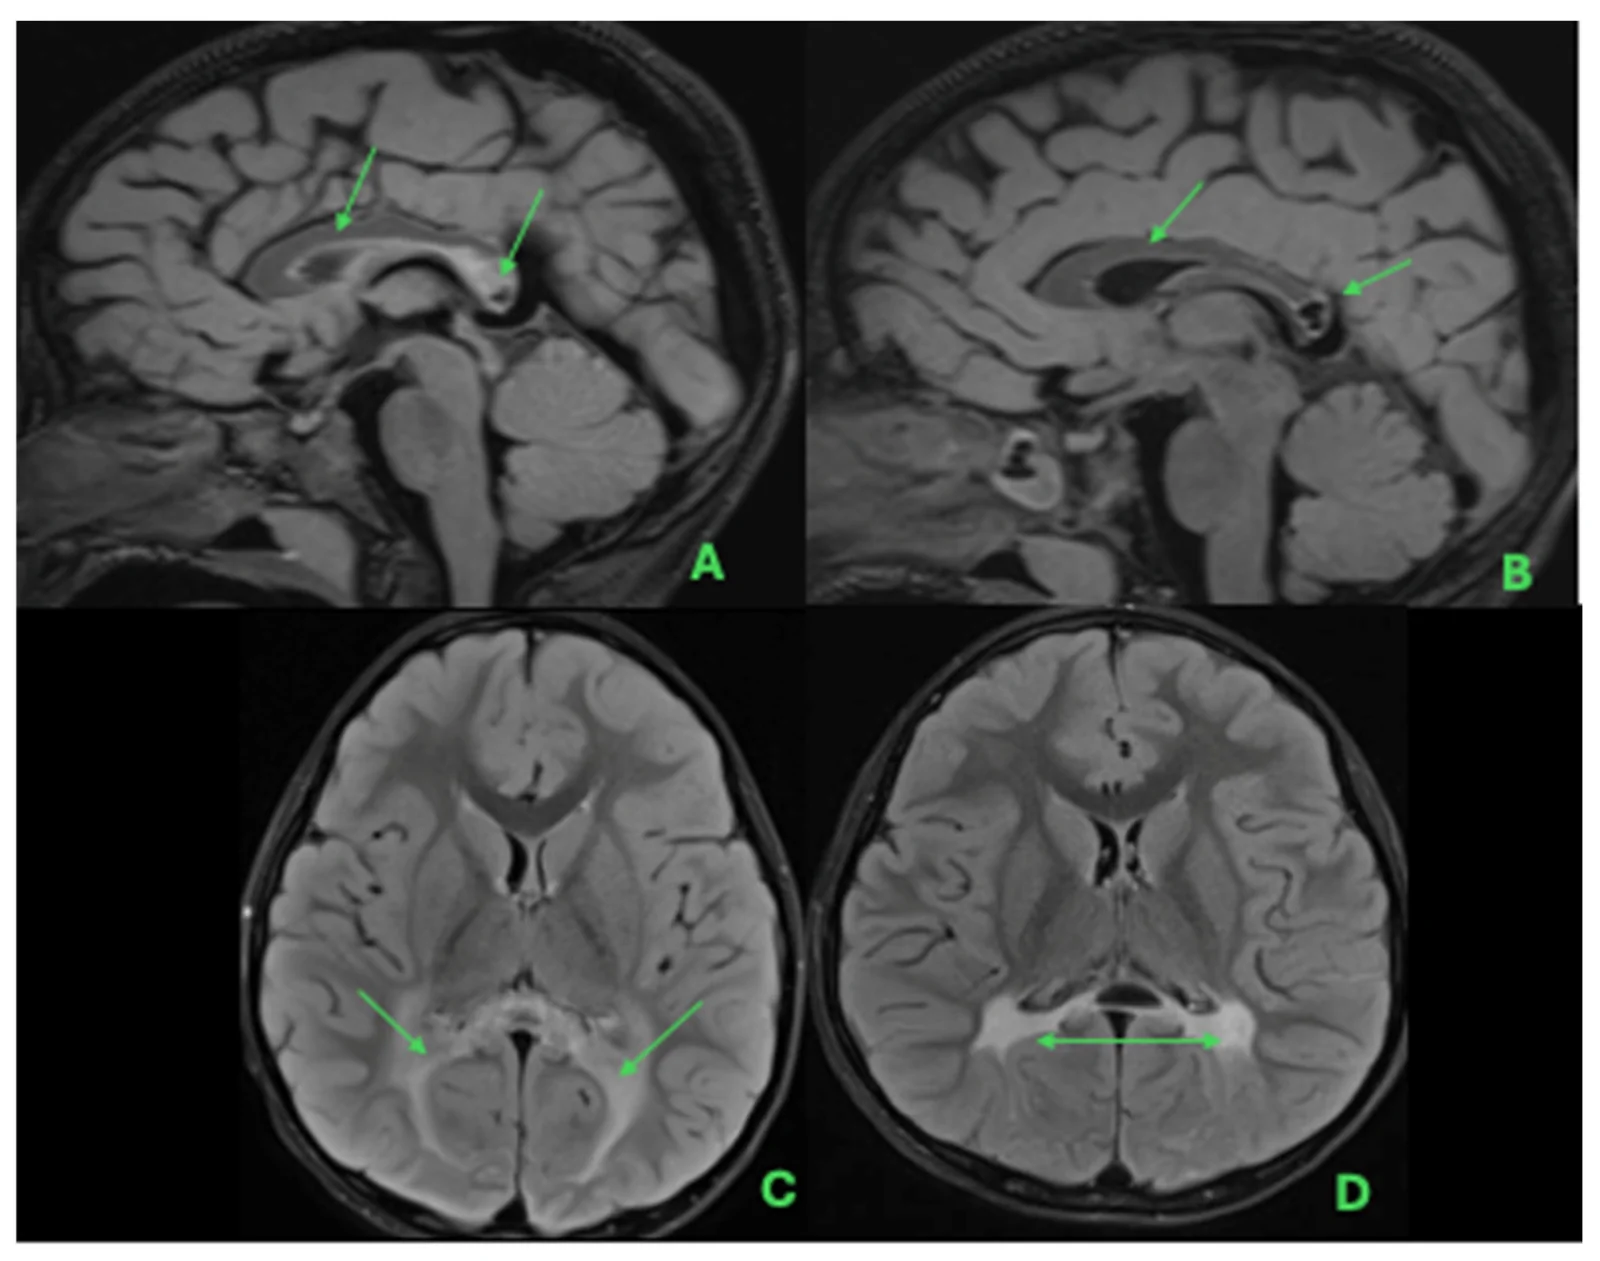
Serial brain MRI demonstrating pre- and post-transplant evolution of white matter abnormalities in a patient with Krabbe disease. Pre-transplant axial T2 FLAIR imaging demonstrates involvement of the splenium of the corpus callosum (A) and symmetric extension of abnormal signal within the bilateral periatrial and parieto-occipital periventricular white matter (C). Follow-up imaging after the second transplant demonstrates stable involvement of the corpus callosum on sagittal FLAIR sequences (B), with persistent but stable periventricular white matter abnormalities on subsequent imaging approximately one year later (D).
